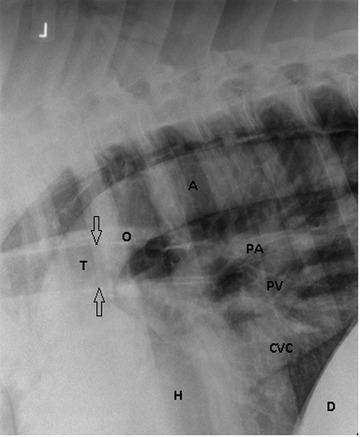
Latero‐lateral radiograph of the craniodorsal thorax; cranial is to the left, image on admission. Due to the presence of air in the mediastinum the outlines of the mediastinal contents such as the oesophagus (O), major vessels (A) aorta, (PA) pulmonary arteries, (PV) pulmonary veins, (CVC) caudal vena cava‐cardiac outlines (black arrows) and outlines of the trachea (T) are abnormally well visualised. A pneumomediastinum was diagnosed. (H) heart, (D) diaphragm
